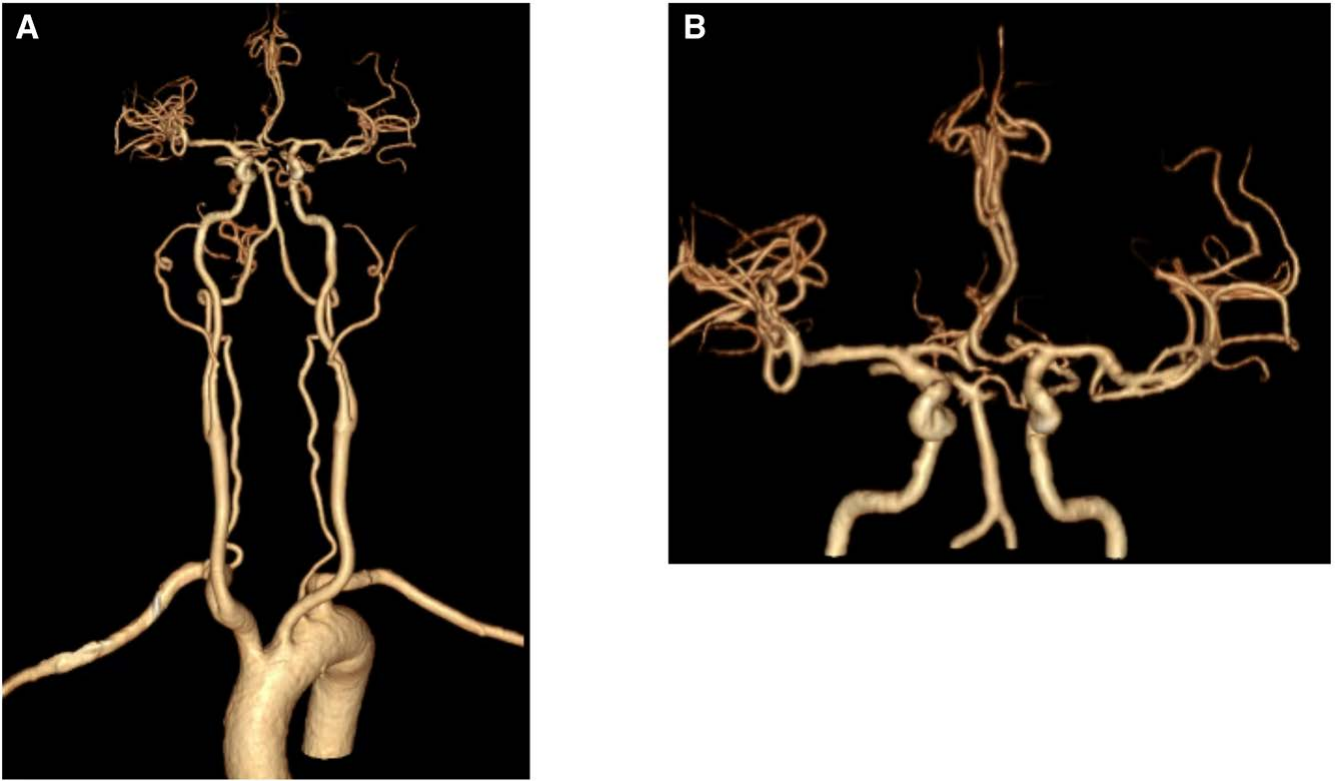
CT angiography performed under craniocervical conditions exhibited no anomalies.
